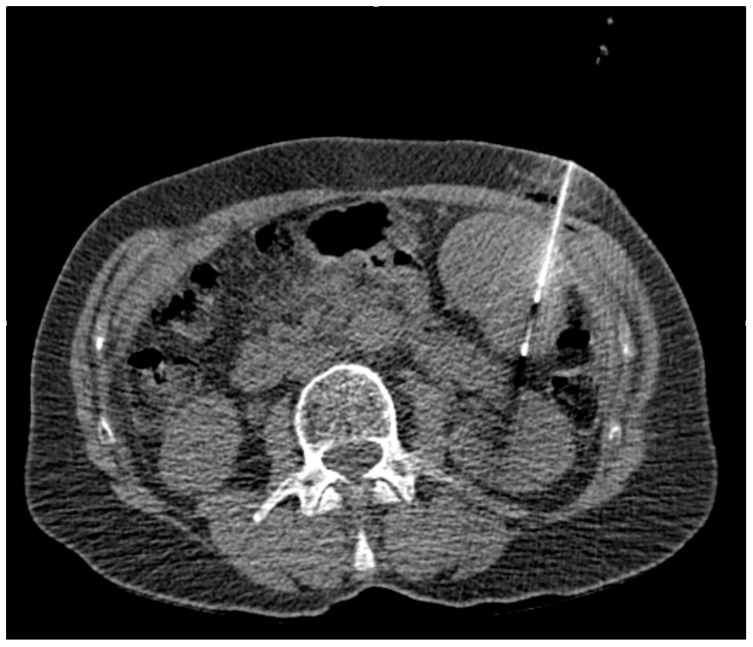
CT-guided spleen biopsy.
